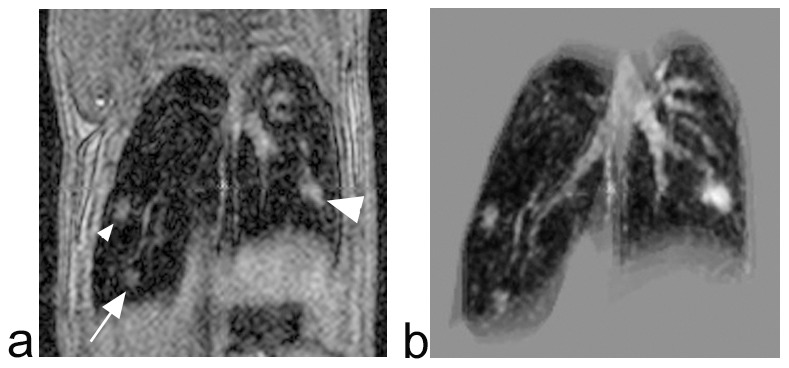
Representative coronal T1-weighted MR images of lung tumors. Two lung tumors located in the right lung (small arrowhead and arrow) and one lung tumor located in the left lung (large arrowhead) (a). Three-dimensional volume-rendered image generated from the 3D data showing the relative locations of tumors and pulmonary vessels (b).
